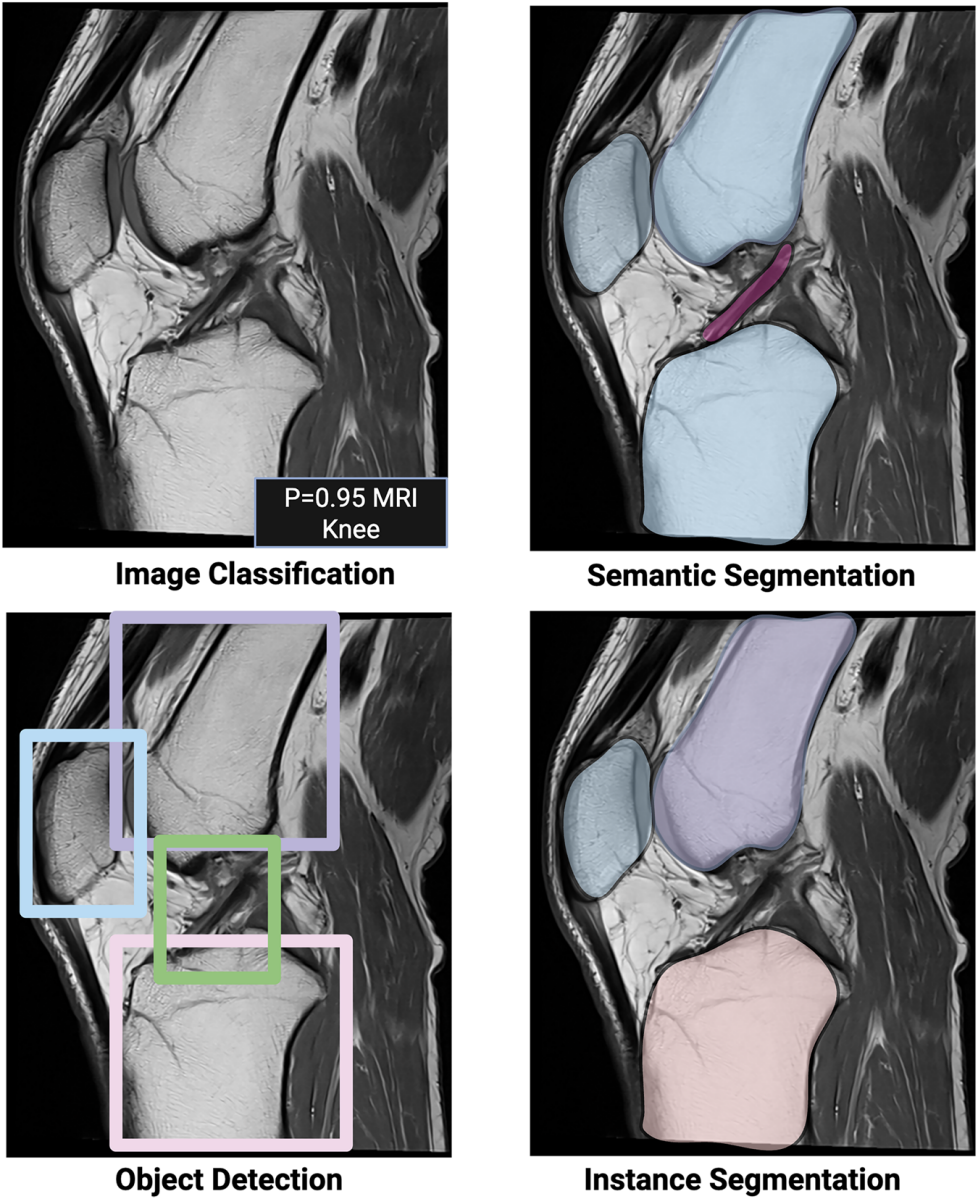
Technical performance: segmentation and object detection methods. Image classification: assigns a single probability score indicating the likelihood of the image belonging to a specific class. In this case, probability (*p*) = 0.95 for class “MRI knee” vs class “MRI hand”. Semantic segmentation: labels each pixel with a class (e.g., bones and ligaments) to create a detailed pixel-level map of the image. Object detection: identifies and localizes structures of interest with bounding boxes around anatomical regions or lesions (e.g., femur, tibia, patella, and anterior cruciate ligament) without providing segmentation. Instance segmentation: combines object detection and segmentation by labeling individual objects within the same class (e.g., the individual structures within class bones) (created with biorender.com)
